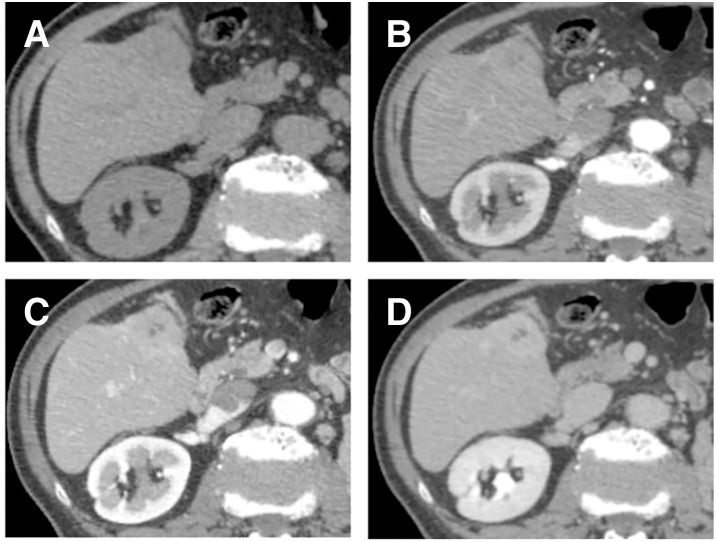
Contrast-enhanced CT imaging of the liver. (A) Plain CT showing a 35-mm mass in segment 5 of the liver. (B) Arterial-phase contrast-enhanced CT showing mild heterogeneous enhancement of the hepatic lesion. (C) Portal venous phase showing gradual progression of the intralesional enhancement, demonstrating a progressive (delayed) enhancement pattern. (D) Delayed enhancement of the lesion in equilibrium-phase CT.
